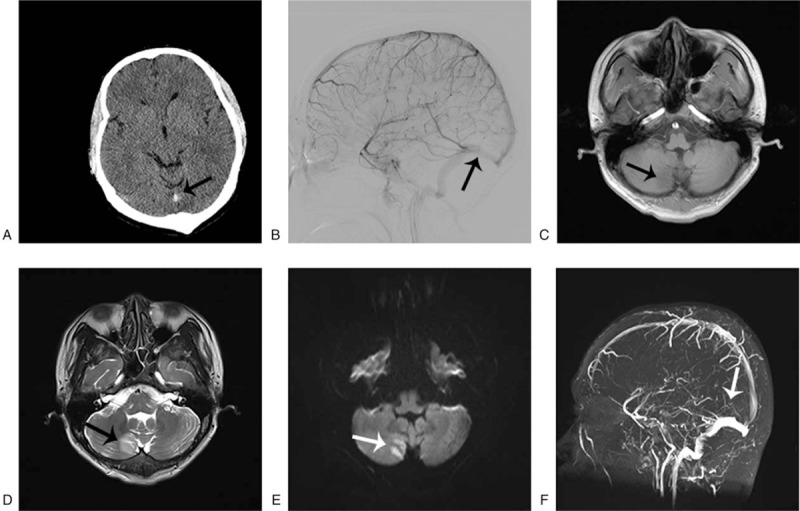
(A) Noncontrast cerebral CT scan showing spontaneous hyperdensity of the straight sinus (black arrow). (B) Straight sinus (black arrow) is not seen on cerebral DSA, confirming thrombosis. (C–E) T1-weighted MRI, T2-weighted MRI and DWI showing an acute infarction at the right cerebellar hemisphere (black arrow). (F) Noncontrast magnetic resonance venography showing the defect in the vein of the straight sinus (white arrow). CT = computed tomography, DSA = digital subtraction angiography, MRI = magnetic resonance imaging.
